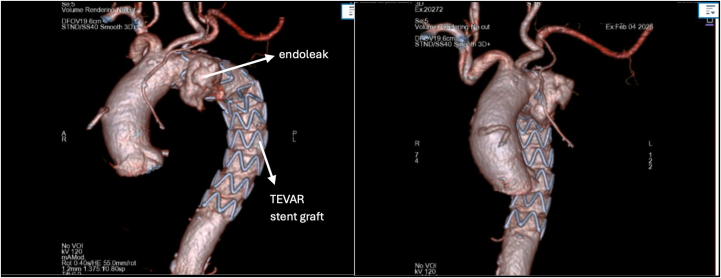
Computed Tomography Angiography Showing an Aortic Aneurysm and TEVAR Stent TEVAR = thoracic endovascular aortic repair.
